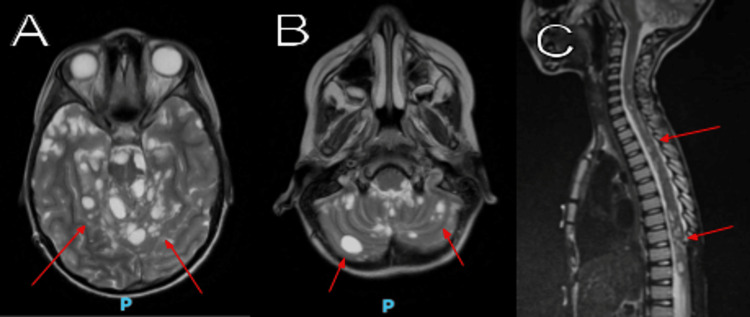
Brain and spine MRI (axial T2 propeller views) demonstrate numerous cystic tumoral lesions (red arrows) throughout the brain (A), cerebellum (B), and spine (C).
